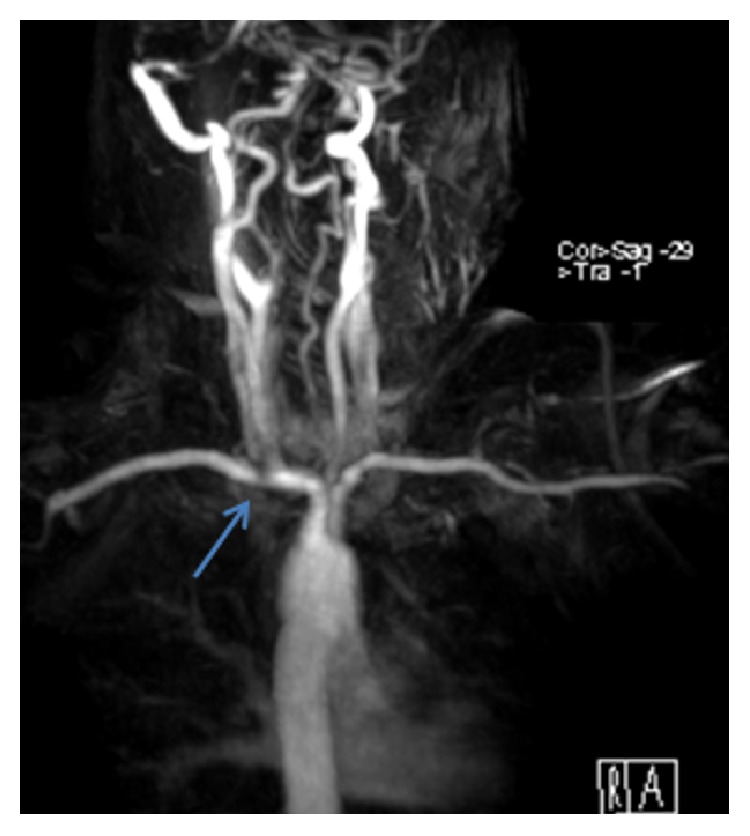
MRI showing compression (arrow) of the right subclavian artery.
